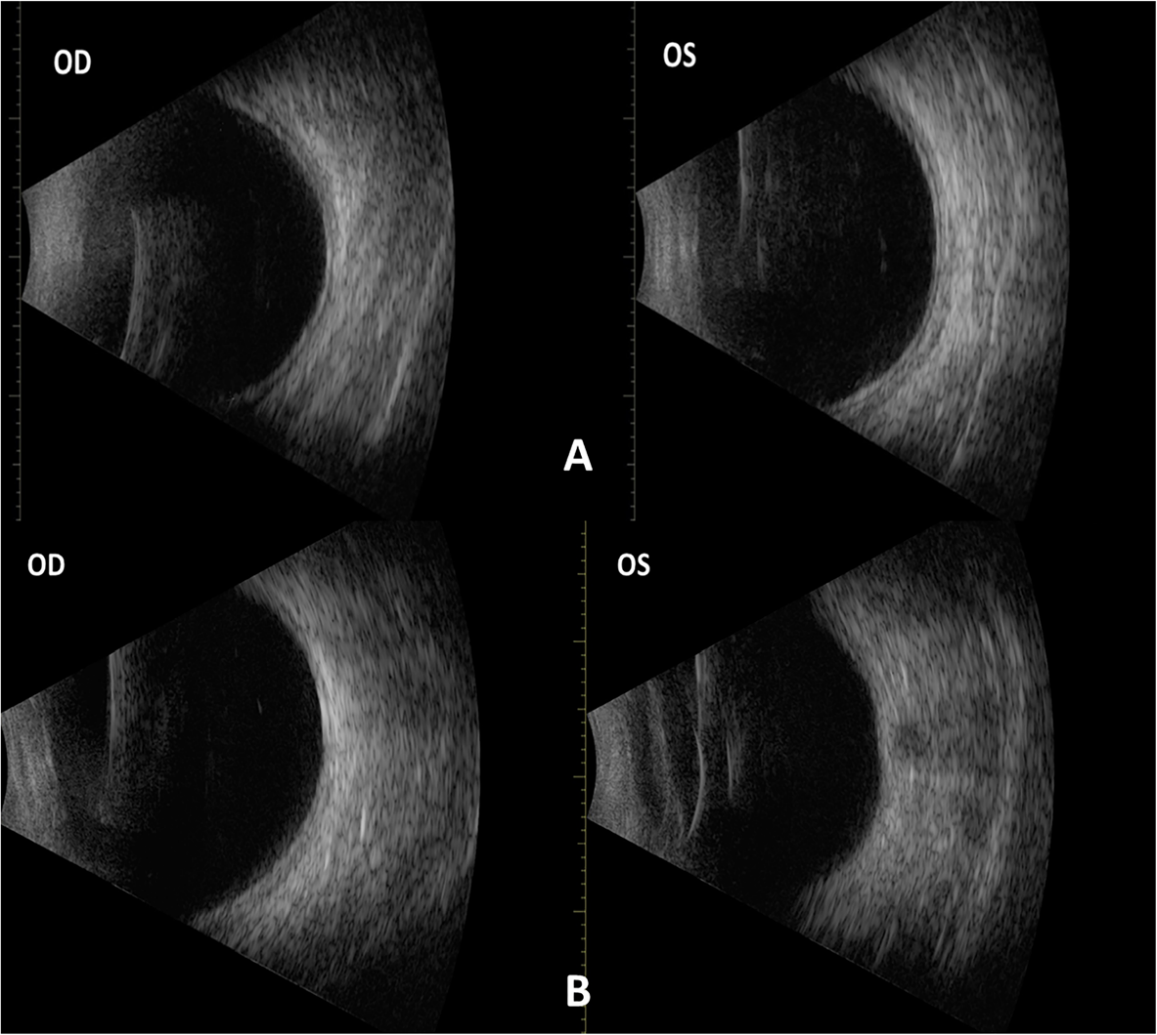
Diffuse choroidal thickening in the both eyes on the first visit (a) and disappearance on control visit (b) in B scan ultrasonography
